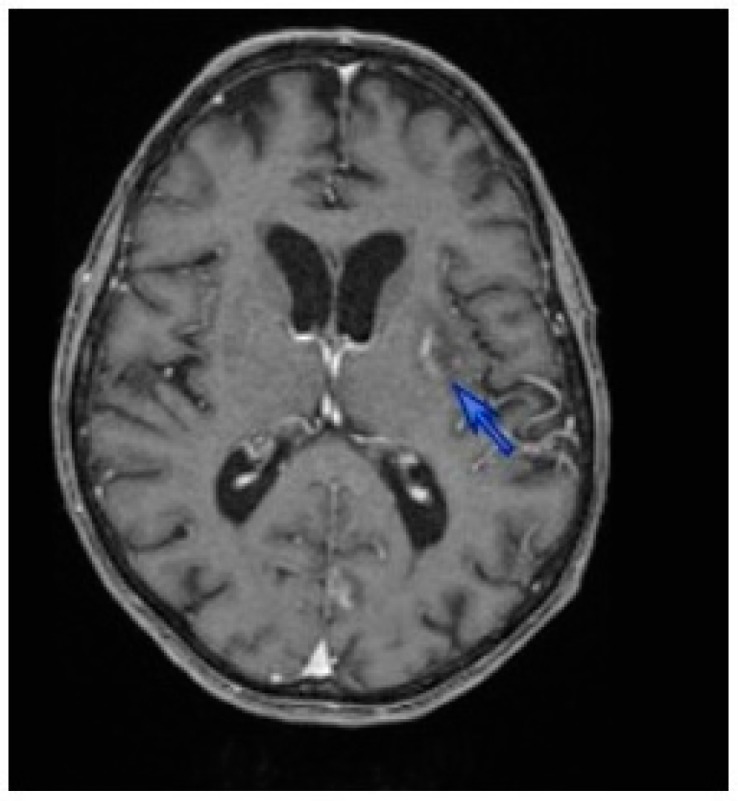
Computer tomography (CT) brain showing a 23 nm lenticular lesion in the left basal ganglia (blue arrow).
